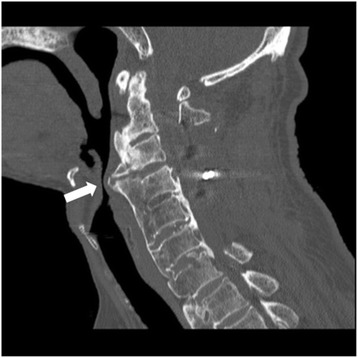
Sagittal CT showing overhang of posterior hypopharyngeal wall by osteophytes (*white arrow*) in a 69-year-old man
